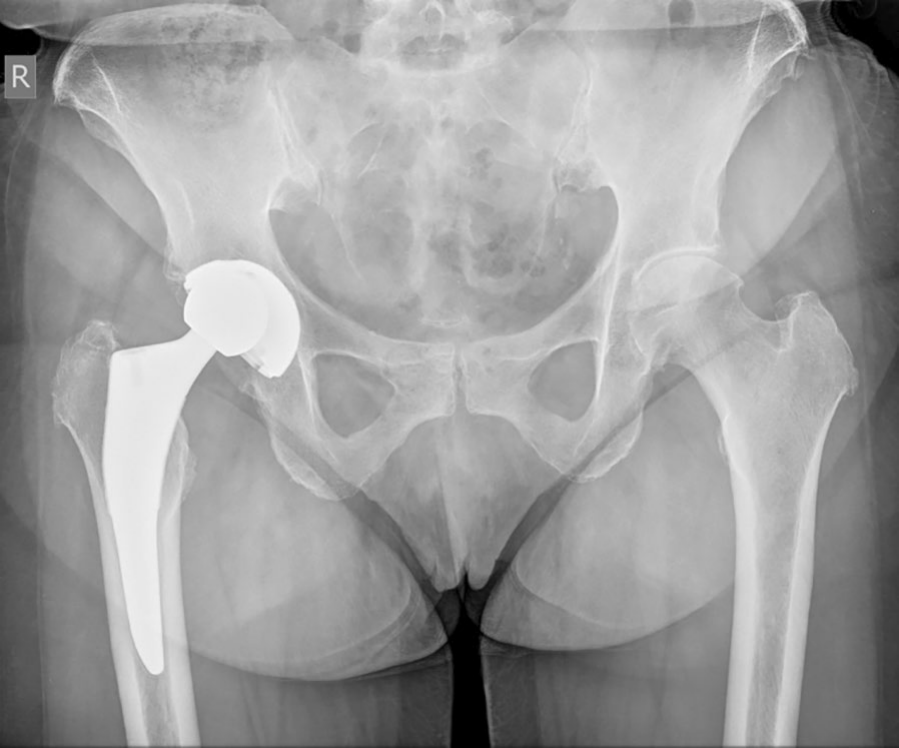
Anteroposterior X-ray of the pelvis showing a dislocation of the femoral head caused by liner dissociation from the acetabular cup. The image clearly depicts the contact of the dislocated femoral head with the acetabular cup
